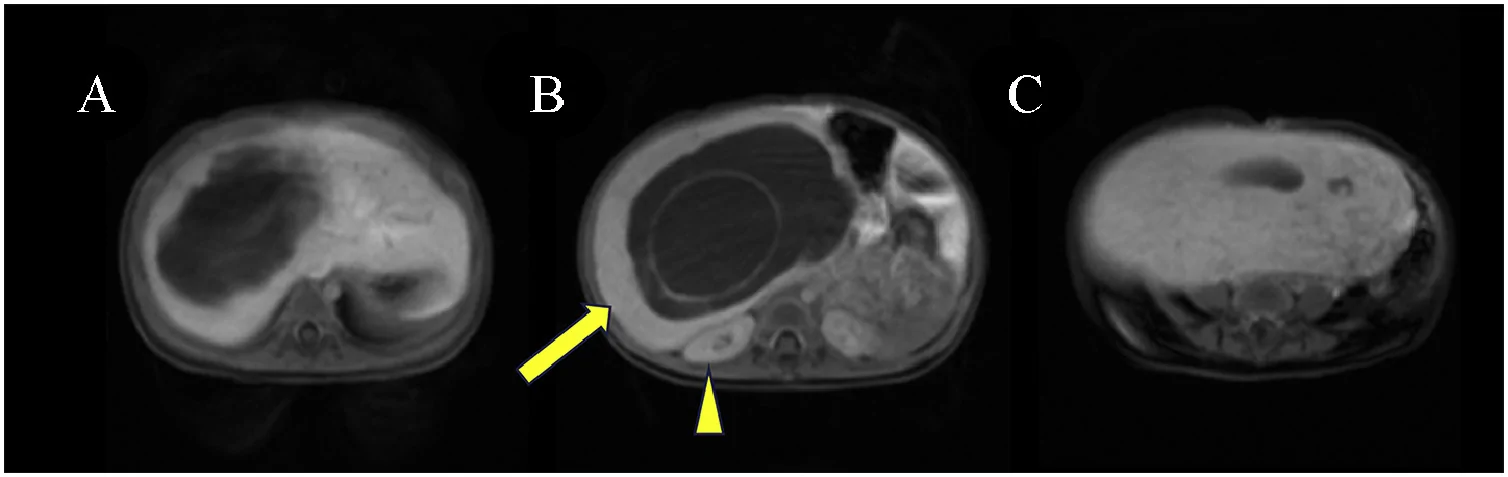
Hepatobiliary-phase gadoxetate disodium–enhanced magnetic resonance imaging. (A-C): Hepatobiliary phase images arrow: solid component; arrowhead: renal cortex. In the hepatobiliary phase, the enhanced areas exhibited lower signal intensity than the normal liver. The solid component shows low signal intensity compared to the renal cortex in precontrast images and exhibits isointense signal in the hepatobiliary phase.
